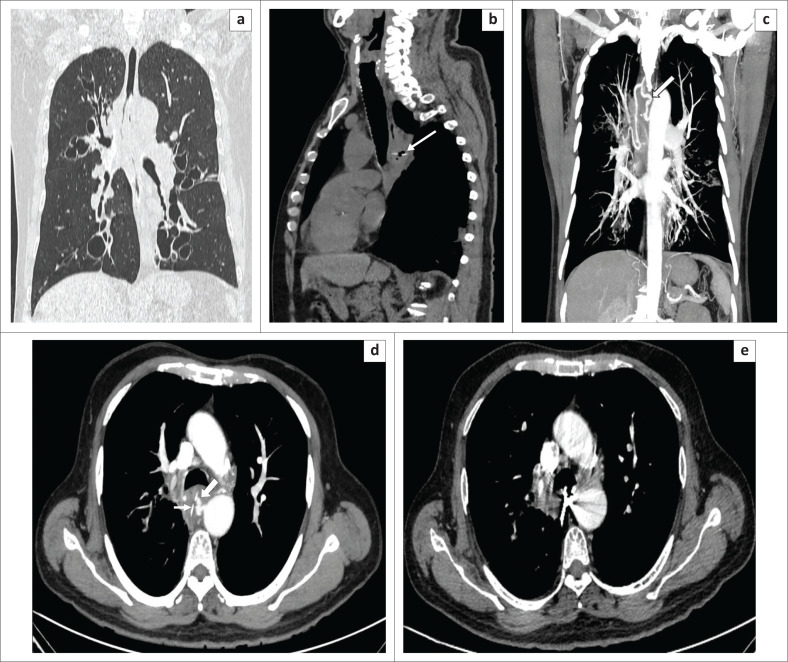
Computed tomography (CT) images of a 61-year-old man with a pseudoaneurysm of a hypertrophied bronchial artery following impaction of a retro-oesophageal fish bone (FB). Reformatted coronal CT images indicate the (a) underlying bronchiectasis (b) impacted retroesophageal FB and collection (thin arrow). (c) Post contrast 3D-multiplanar reformation (MPR) coronal image shows the hypertrophied bronchial artery (thick arrow). (d) Post contrast 3D CT axial image shows the impacted FB (thin arrow) causing a pseudoaneurysm of the hypertrophied bronchial artery (thick arrow). (e) Post embolisation of the bronchial artery pseudoaneurysm; the FB was removed.
